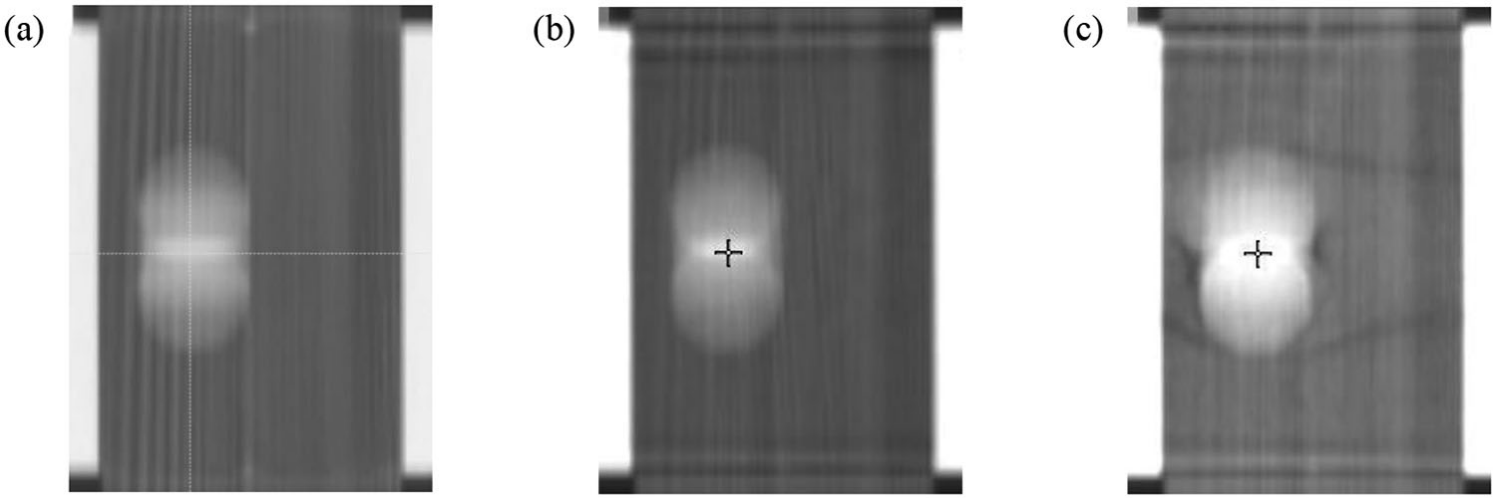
The spherical target imaged with (a) Sim CT, (b) 60‐second CBCT, and (c) 6‐second CBCT for a regular breathing pattern with a 4‐s period and 13 mm amplitude.
